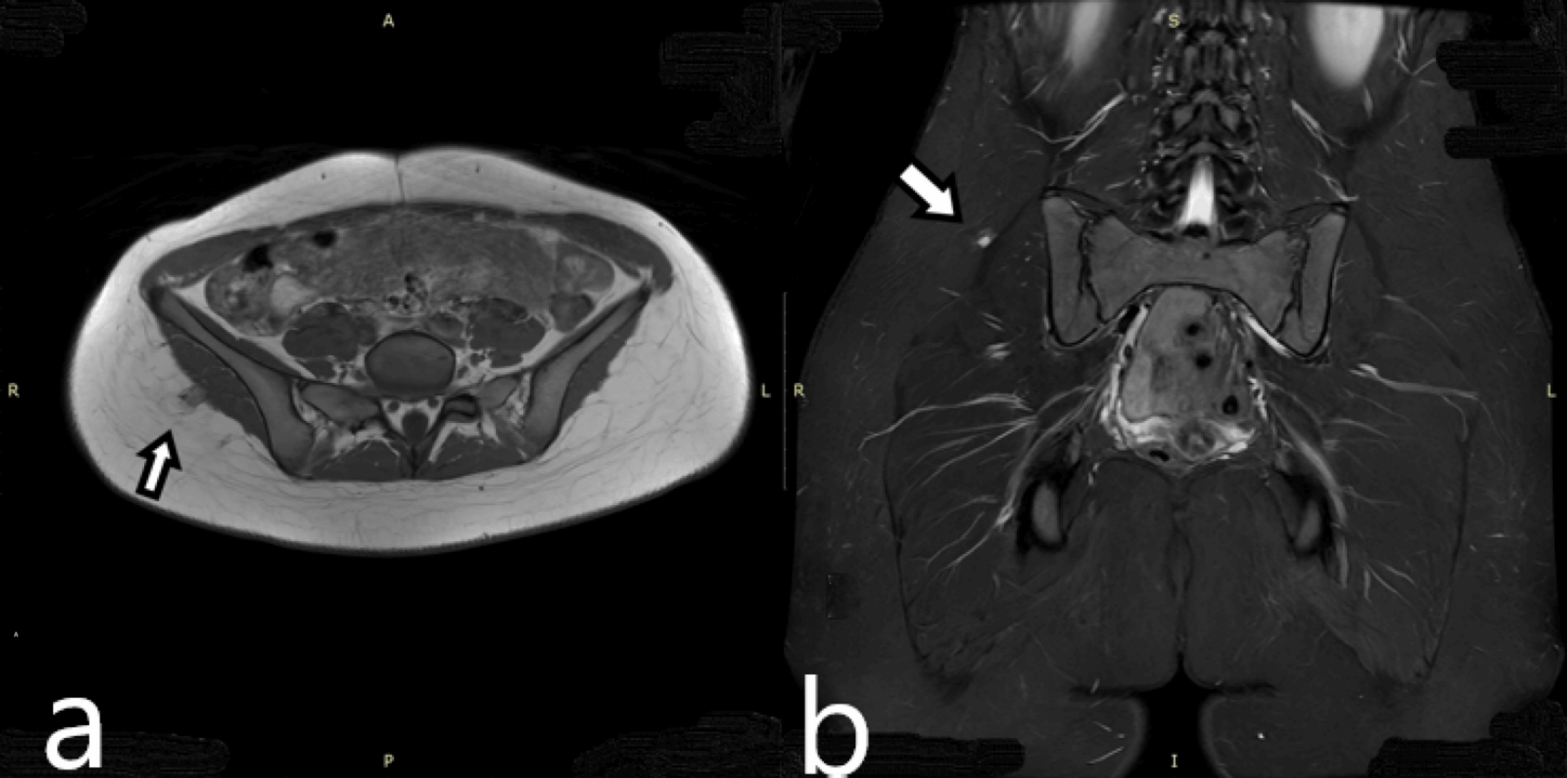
Axial contrast-enhanced T1W MRI and coronal FSE STIR images, after six months from the initial MRI, show a small lesion measuring 0.9 × 0.8 cm, with normal appearance of the surrounding muscles (a) Axial contrast-enhanced T1W MRI. (b) Coronal FSE STIR. FSE, fast spin echo
